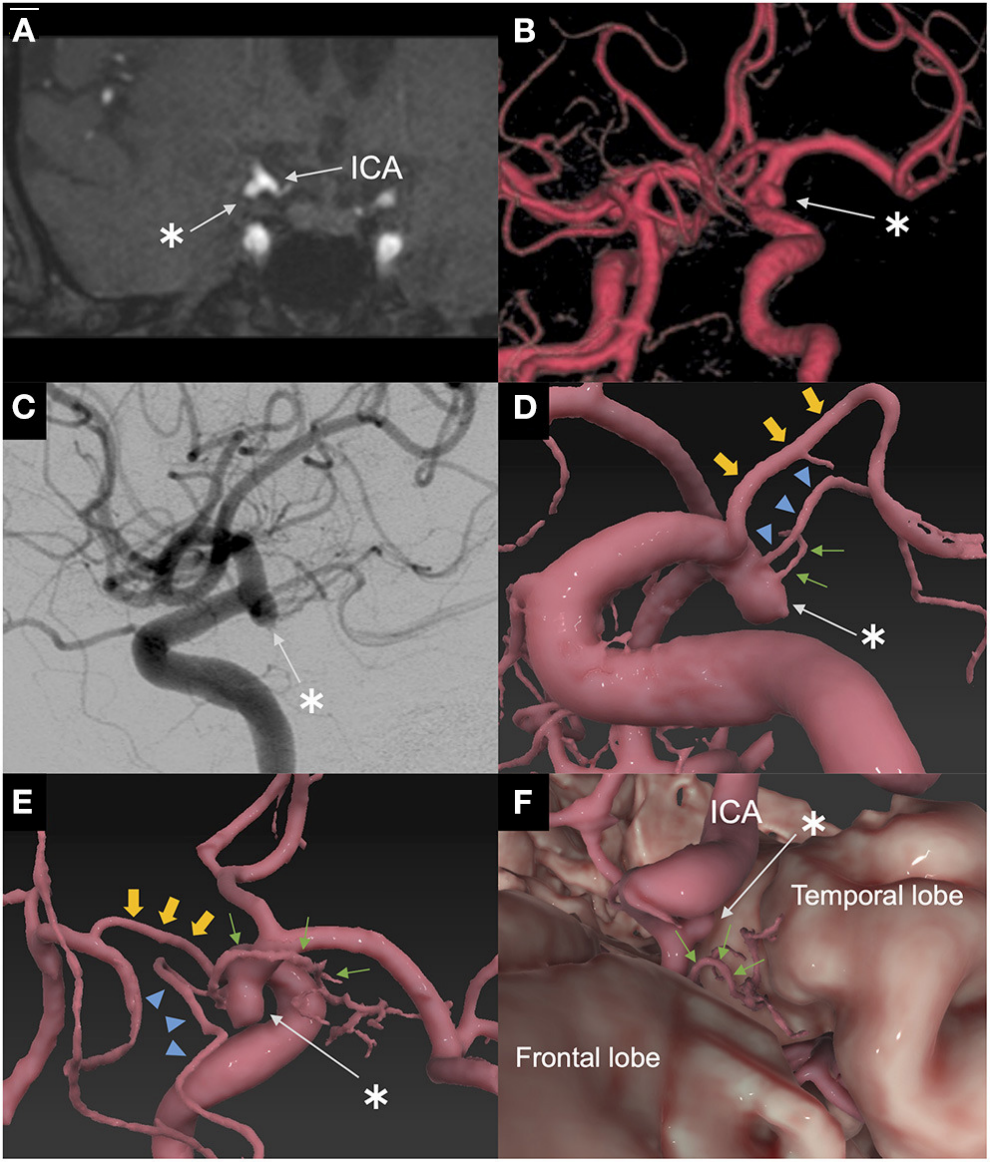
Preoperative imaging. (A) Coronal plane of time-of-flight magnetic resonance angiography on admission. Coronal plane of time-of-flight magnetic resonance angiography showed an unruptured aneurysm of the right internal carotid artery in contact with the temporal lobe. *: aneurysm. (B) Three-dimensional time-of-flight magnetic resonance angiography on admission. Three-dimensional time-of-flight magnetic resonance angiography showed an unruptured aneurysm of the right internal carotid artery supraclinoid portion. *: aneurysm. (C) Lateral view of digital subtraction angiography of the right internal carotid artery. Lateral view of digital subtraction angiography of the right internal carotid artery showing an aneurysm associated with a duplicated middle cerebral artery and anterior choroidal artery. *: aneurysm. (D) Fusion three-dimensional computer graphics integrating MR imaging/MR angiography and three-dimensional rotational angiography. Fusion three-dimensional computer graphics showed that the duplicated middle cerebral artery and the anterior choroidal artery originated from the dome. *: aneurysm; small arrow (green): duplicated middle cerebral artery; arrowhead (blue): anterior choroidal artery; large arrowhead (yellow): posterior communicating artery. (E) Fusion three-dimensional computer graphics. Fusion three-dimensional computer graphics shows that the duplicated middle cerebral artery passed through the sylvian fissure along the M1 segment of the MCA. *: aneurysm; small arrow (green): duplicated middle cerebral artery; arrowhead (blue): anterior choroidal artery; large arrowhead (yellow): posterior communicating artery. (F) Fusion three-dimensional computer graphics. Fusion three-dimensional computer graphics showed that the duplicated middle cerebral artery perfused the anterior temporal lobe. *: aneurysm; small arrow (green): duplicated middle cerebral artery. Fusion three-dimensional computer graphics were reconstructed by GRID 1.1 software (Kompath Inc., Tokyo, Japan).
